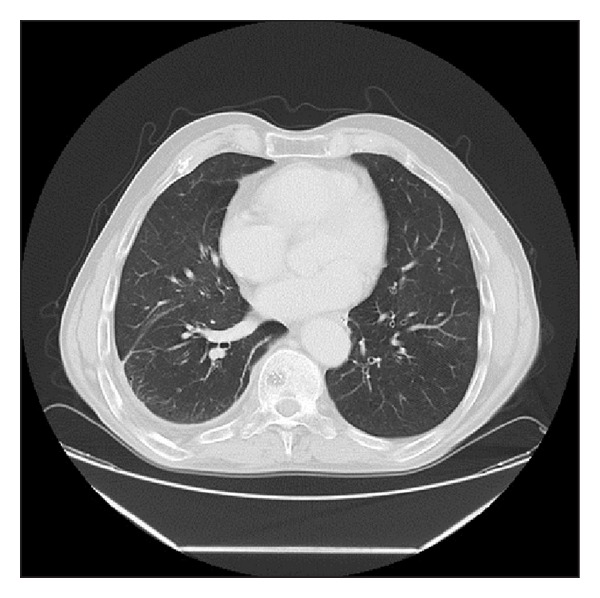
Chest CT revealing a large pulmonary AVM in the basal segmento flowe right lobe.
